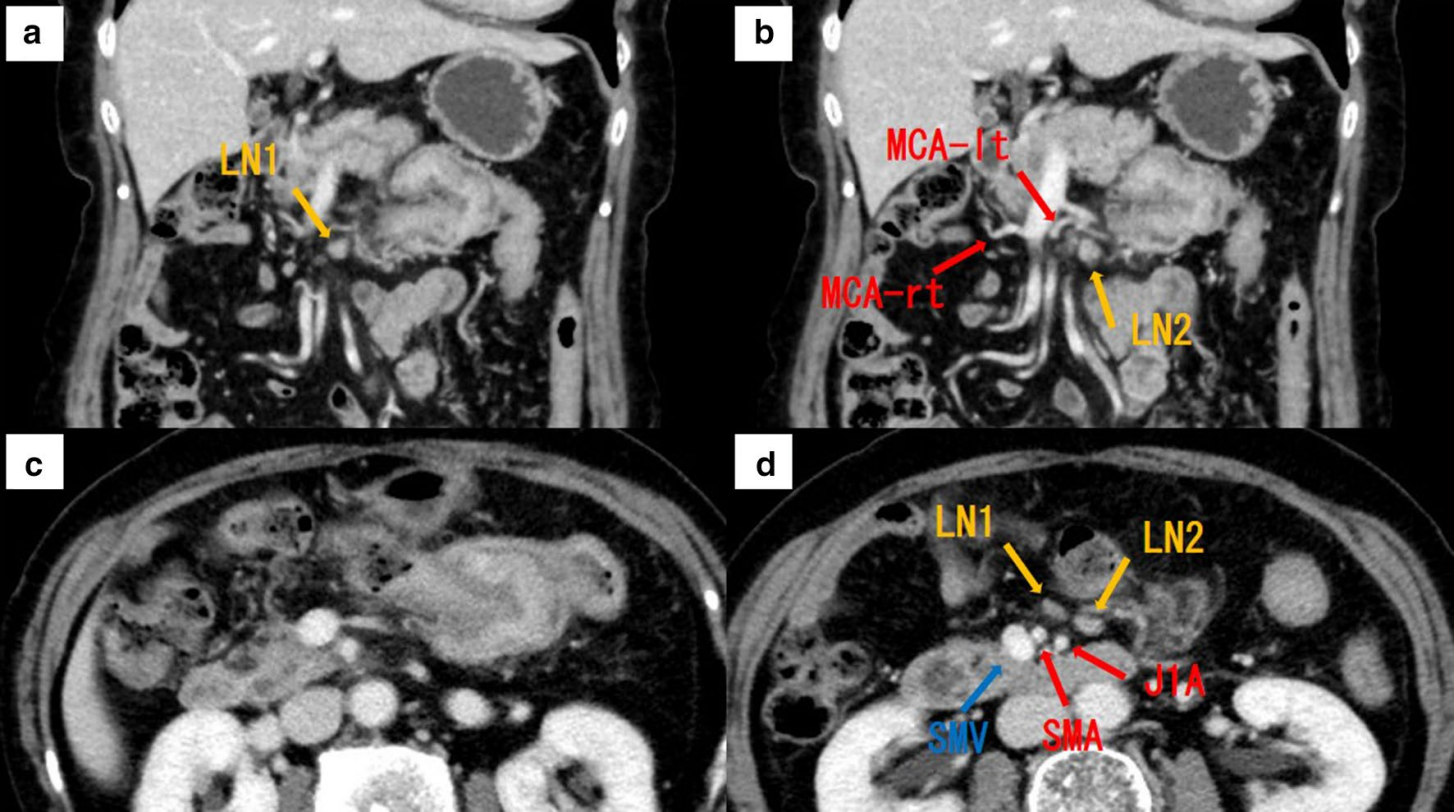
Abdominal contrast CT findings. a The tumor region (approximately 5 cm) with contrast effect was found to be invaginated into the anal intestinal tract on the left side of the transverse colon, but with no visible serosal invasion. b Swollen regional lymph nodes (approximately 10 mm) were found along the root and left branch of the middle colic artery. LN, lymph node; MCA-lt, left branch of middle colic artery; MCA-rt, right branch of middle colic artery; SMA, superior mesenteric artery; SMV, superior mesenteric vein; J1A, jejunal 1st artery
